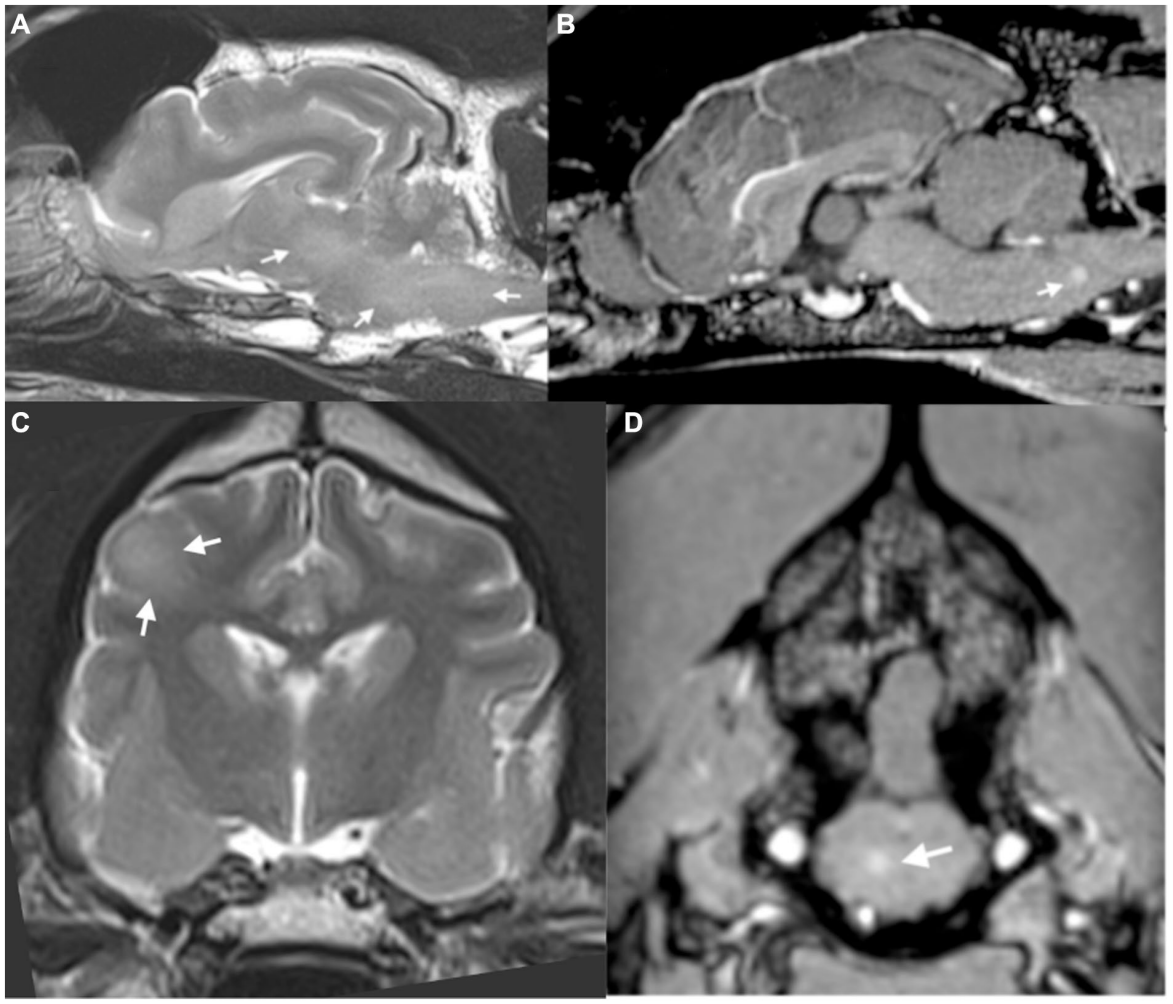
(A) Parasagittal T2-weighted image of the brain, showing diffuse T2-weighted hyperintensity within the brainstem (arrows). (B) Sagittal plane post-contrast T1-weighted image showing focus of contrast enhancement within the medulla (arrows). (C) Transverse plane T2-weighted image of the brain at the level of the interthalamic adhesion showing diffuse T2-weighted hyperintensity within the right parietal lobe (arrows). (D) Transverse post-contrast T1-weighted image at the level of the caudal cerebellar vermis showing small focus of contrast enhancement within the brainstem (arrow).
